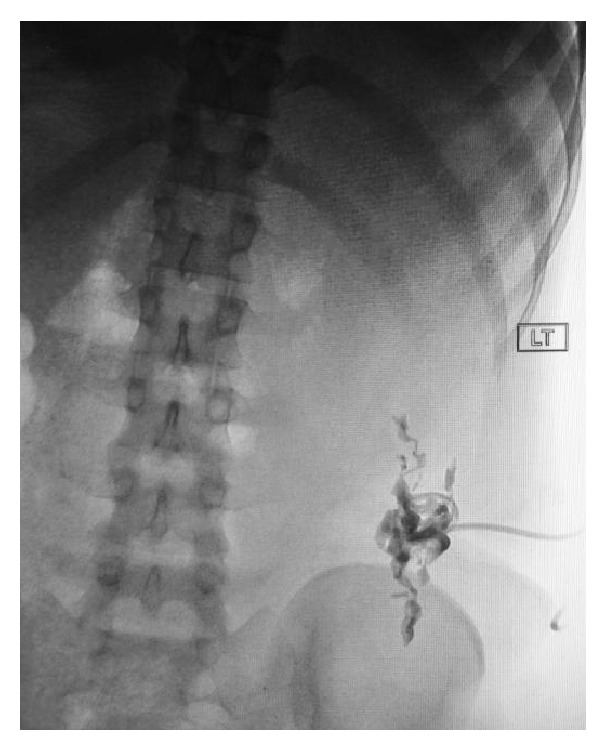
X-ray fluoroscopy confirming placement of colonic abscess drain.
